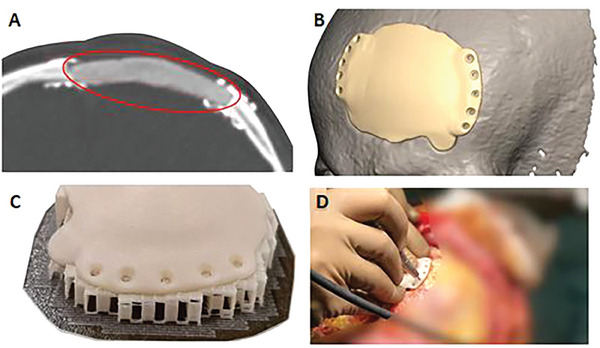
Personalized maxillofacial osteosynthesis system: A) computed tomography image of a frontal lobe defect temporarily filled with neurosurgical cement, B) reconstruction of the patient's skull and the creation of a three‐dimensional implant model fitted to the defective bone, C) 3D‐printed implant manufactured of poly(l‐lactide‐*co*‐d,l‐lactide) and nanohydroxyapatite formulation, and D) surgical procedure of a scaffold implantation in the patient's skull. Reprinted from [www.cyberbone.eu].
